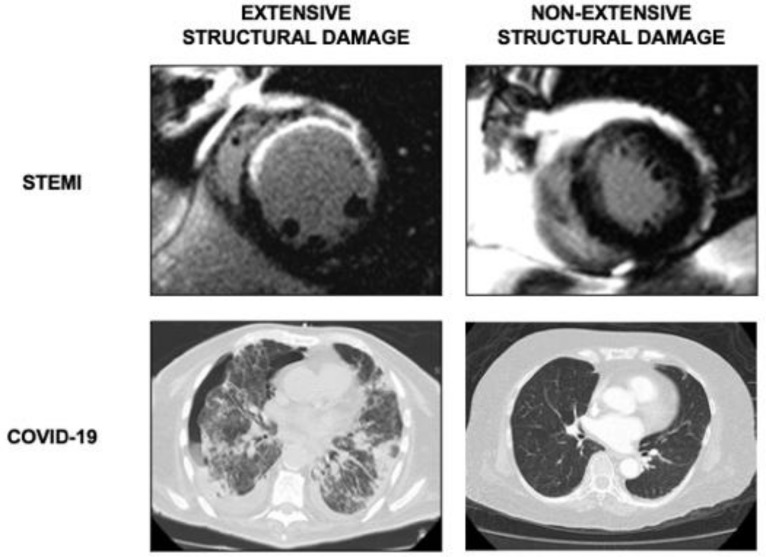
Extension of structural damage. In STEMI patients (upper panel), CMR images of extensive (left) and non-extensive (right) infarct size. In COVID-19 patients (lower panel), CT studies of bilateral (left) and unilateral (right) pneumonia. CMR: cardiac magnetic resonance. COVID-19: coronavirus disease 2019. CT: computed tomography. STEMI: ST-segment elevation myocardial infarction.
